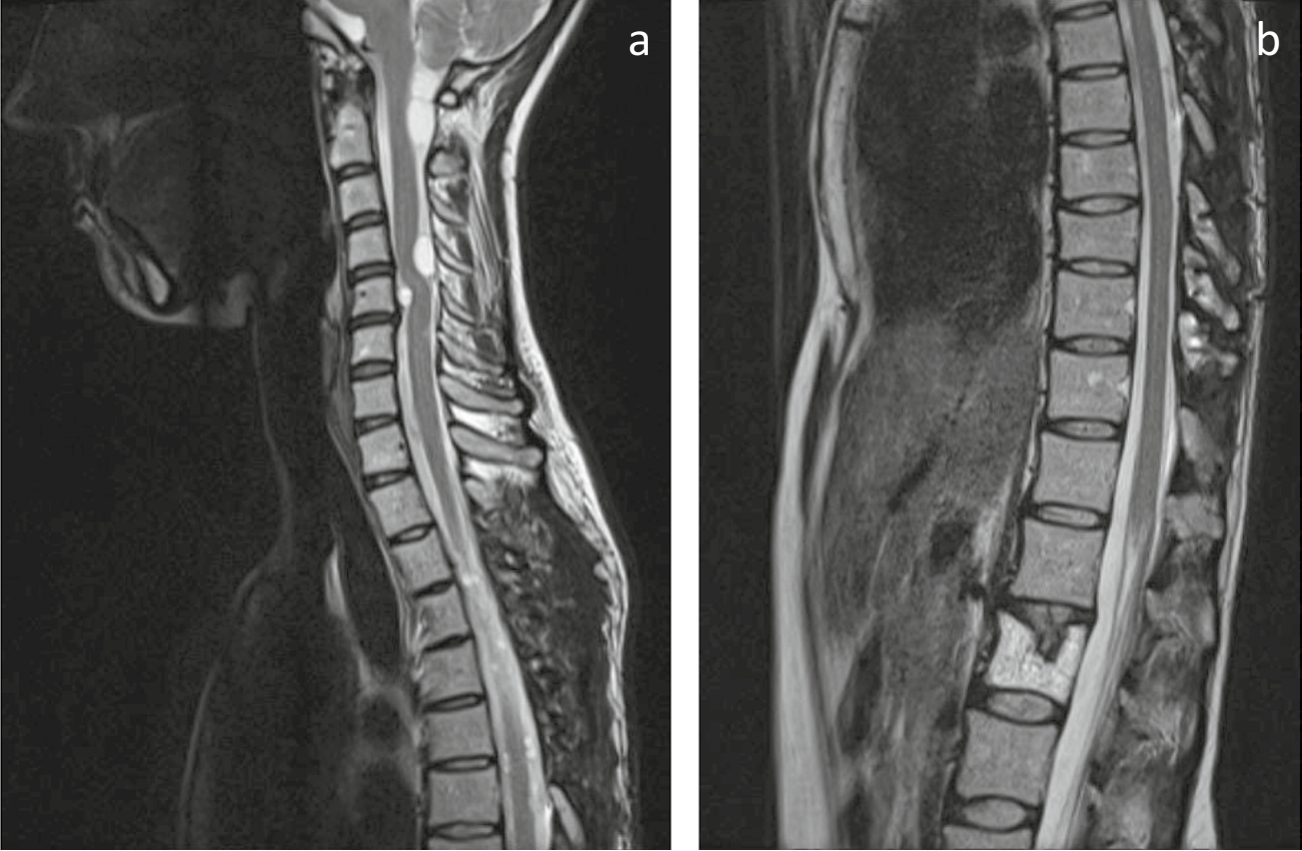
Sagittal MRI sections of the a cervical and upper thoracic and b lower thoracic spine after the second surgery performed at Th8–10
